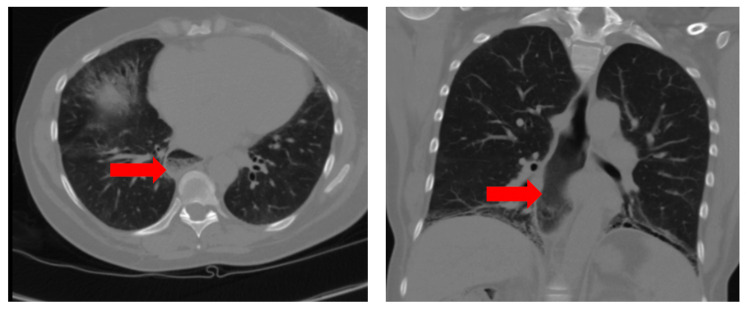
CT сhest demonstrating dilation of the esophagus filled with debris (red arrows) in axial section (left) and coronal section (right)
